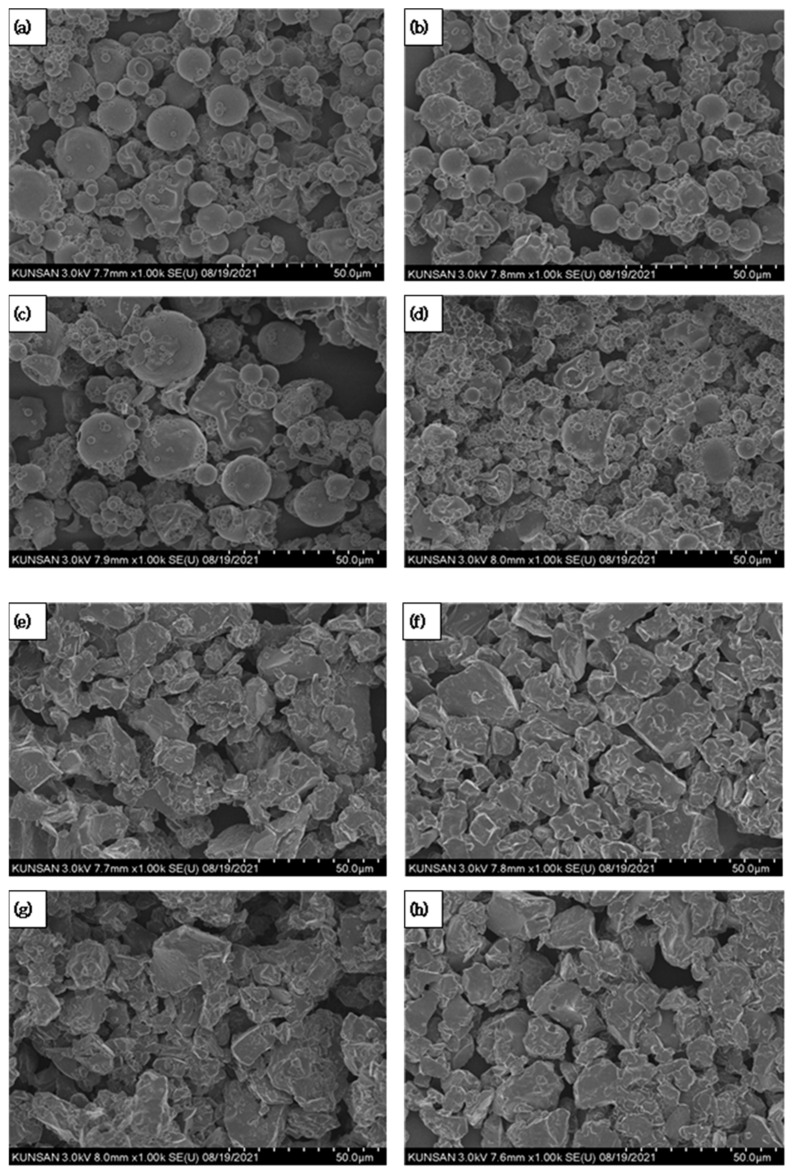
FE-SEM microphotographs of Yuja dried gels: SDG-Y-NE/MD (a), SDG-Y-NE/MD/βCD (b), SDG-Y-NLC/MD (c), SDG-Y-NLC/MD/βCD (d), FDG-Y-NE/MD (e), FDG-Y-NE/MD/βCD (f), FDG-Y-NLC/MD (g), and FDG-Y-NLC/MD/βCD (h).
